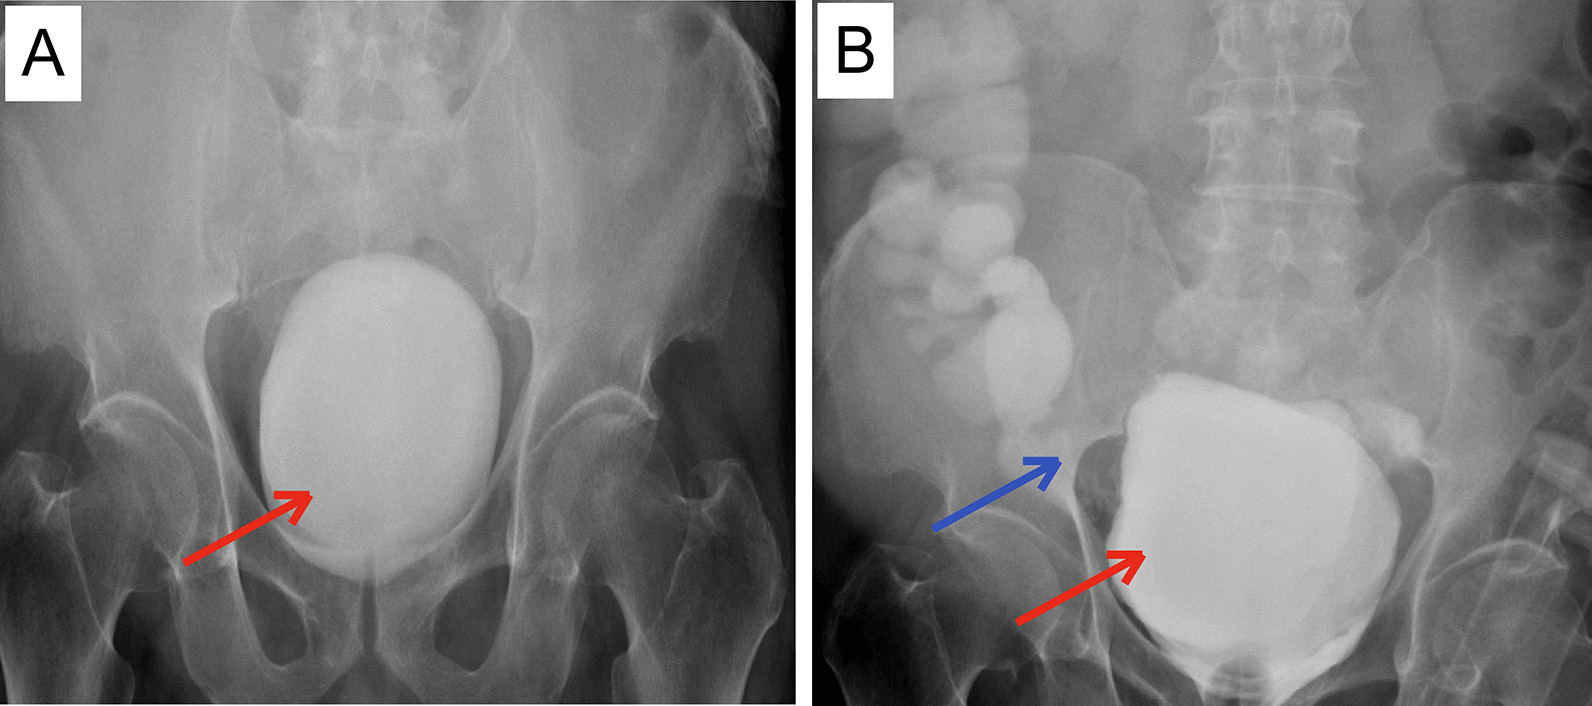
Pelvic X-rays before (A) and after (B) meglumine angiography. Notes: The red arrows in the X-film A and B both indicate giant stones. After injecting a meglumine contrast agent, the contrast material entered the adjacent small intestine and colon, indicated by the blue arrow
